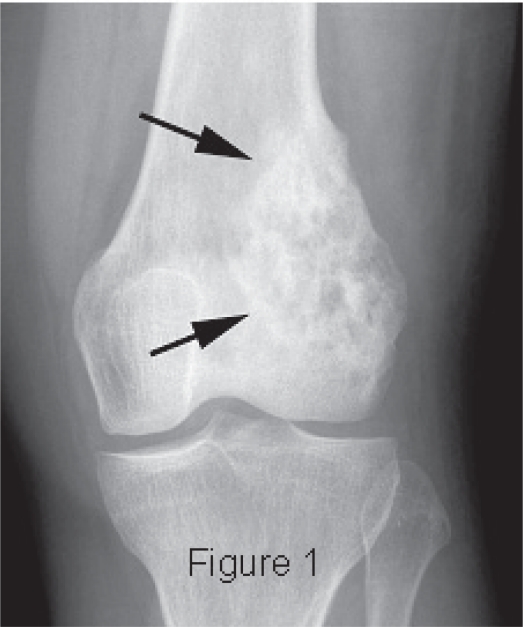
Radiograph showing an osteolytic and osteoblastic intra-medullary tumor characteristic of osteosarcoma.
